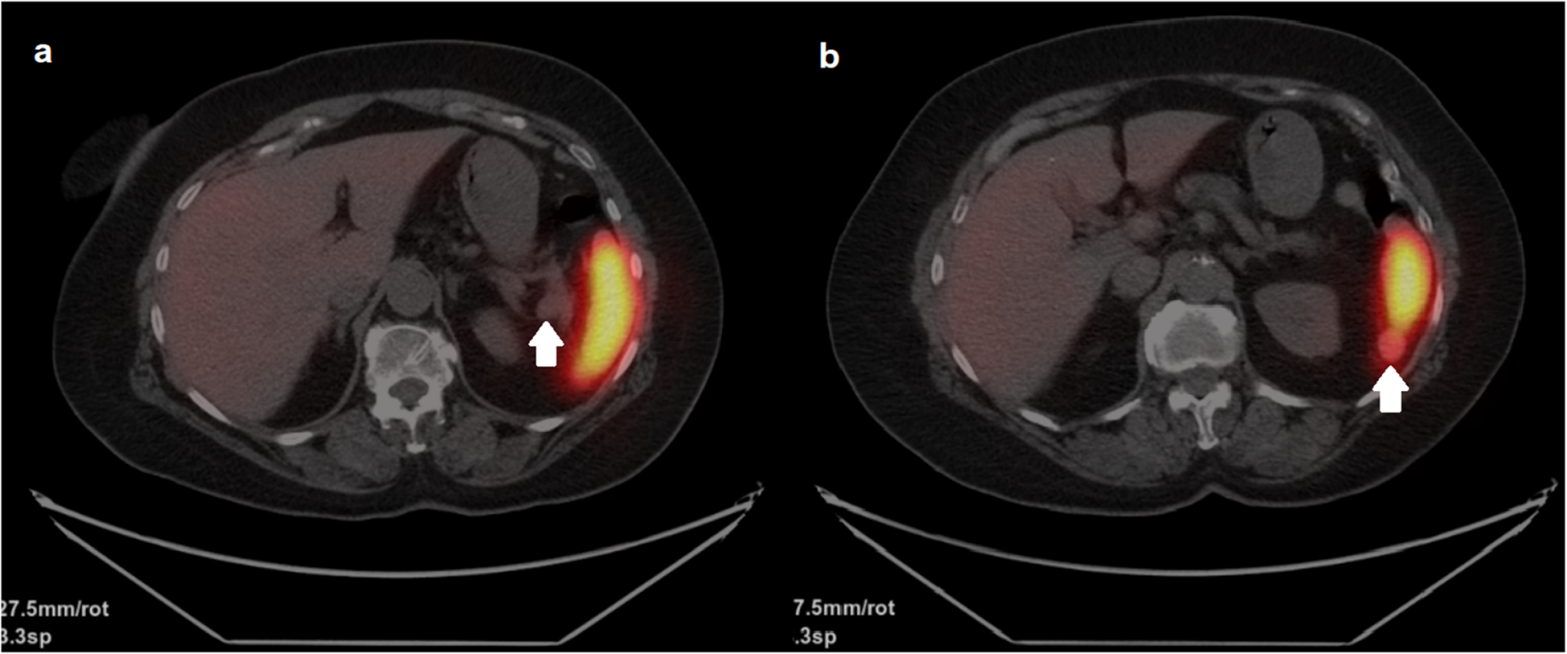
99mTc-HDRBC scintigraphy images, showing no tracer uptake in the pancreatic tail/splenic hilum (a), but remaining tracer uptake in the inferior pole of the spleen (b)
